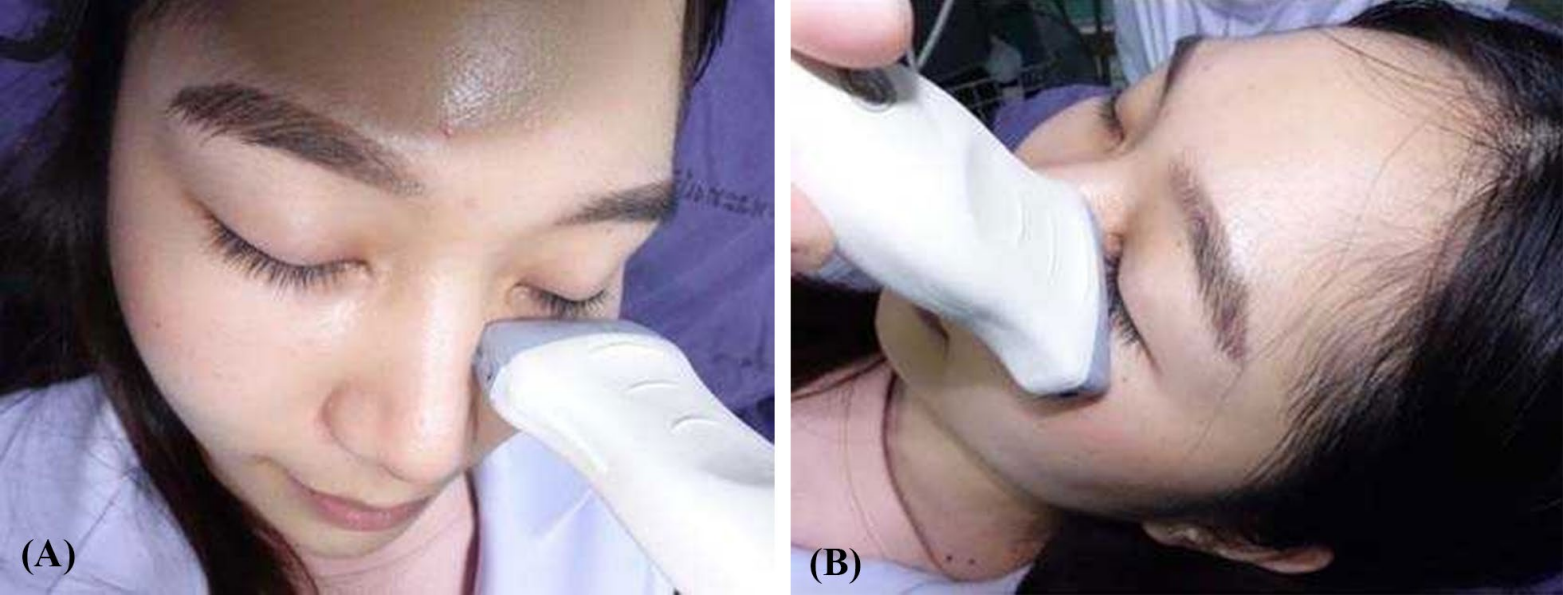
Demonstrating the use of linear-probe ultrasound examination of infra orbital rim. A and B Demonstration using ultrasound for infraorbital rim examination
